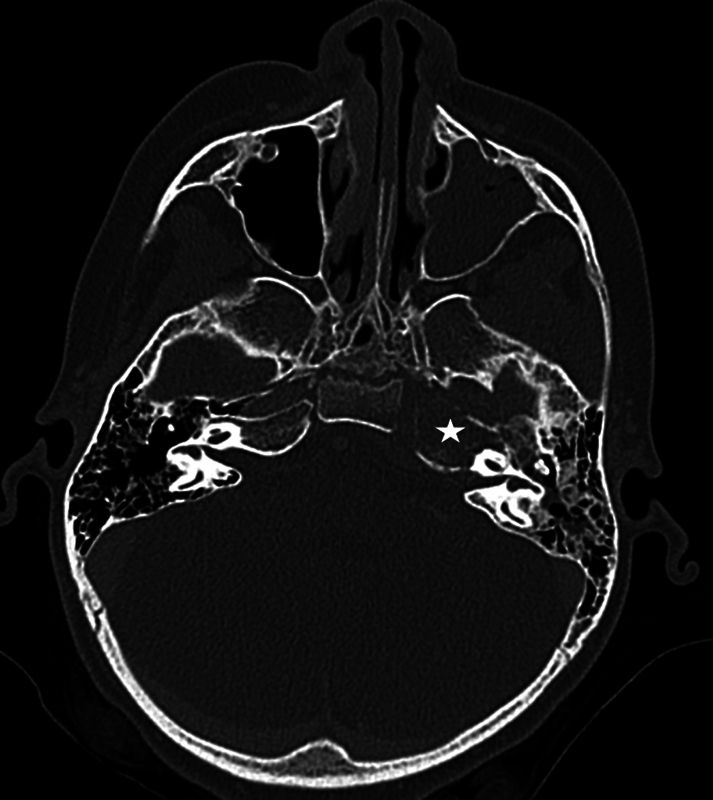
CT temporal bone demonstrating expansile lesion with bony destruction at the left petrous apex (star). There was left maxillary opacification and partial mastoid effusion.
